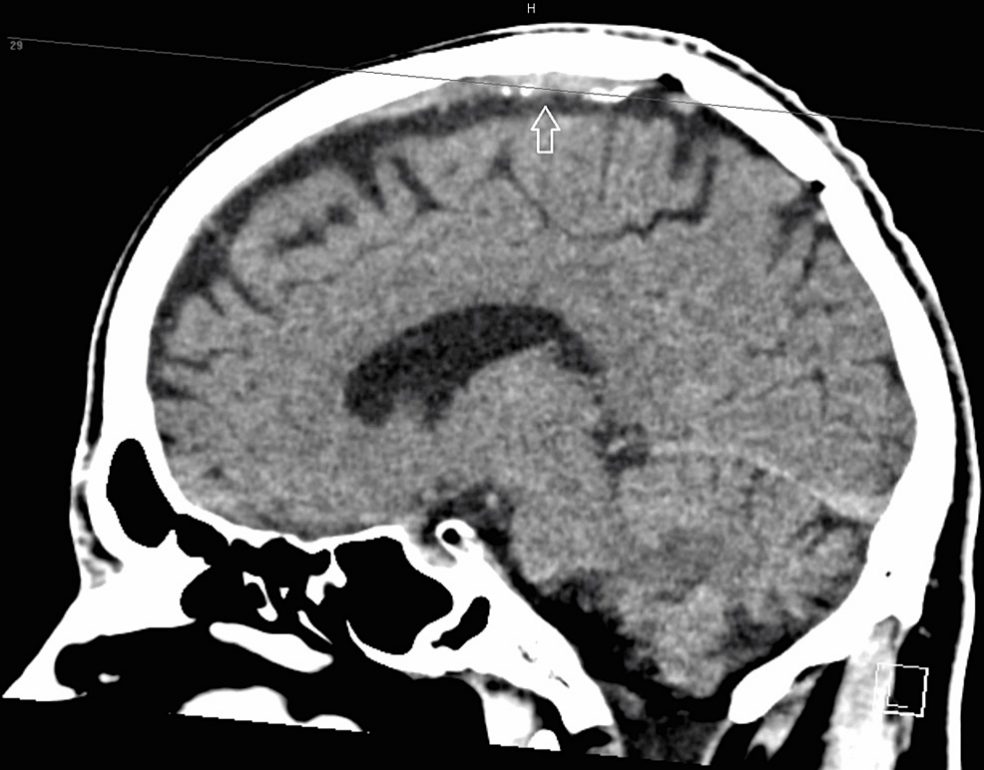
Repeated brain CT scan (sagittal view) showing no sign of bleeding or obvious infarction. Hyperdensity (arrow) due to thrombosed vain at the cortical convexity. CT: computed tomography.
